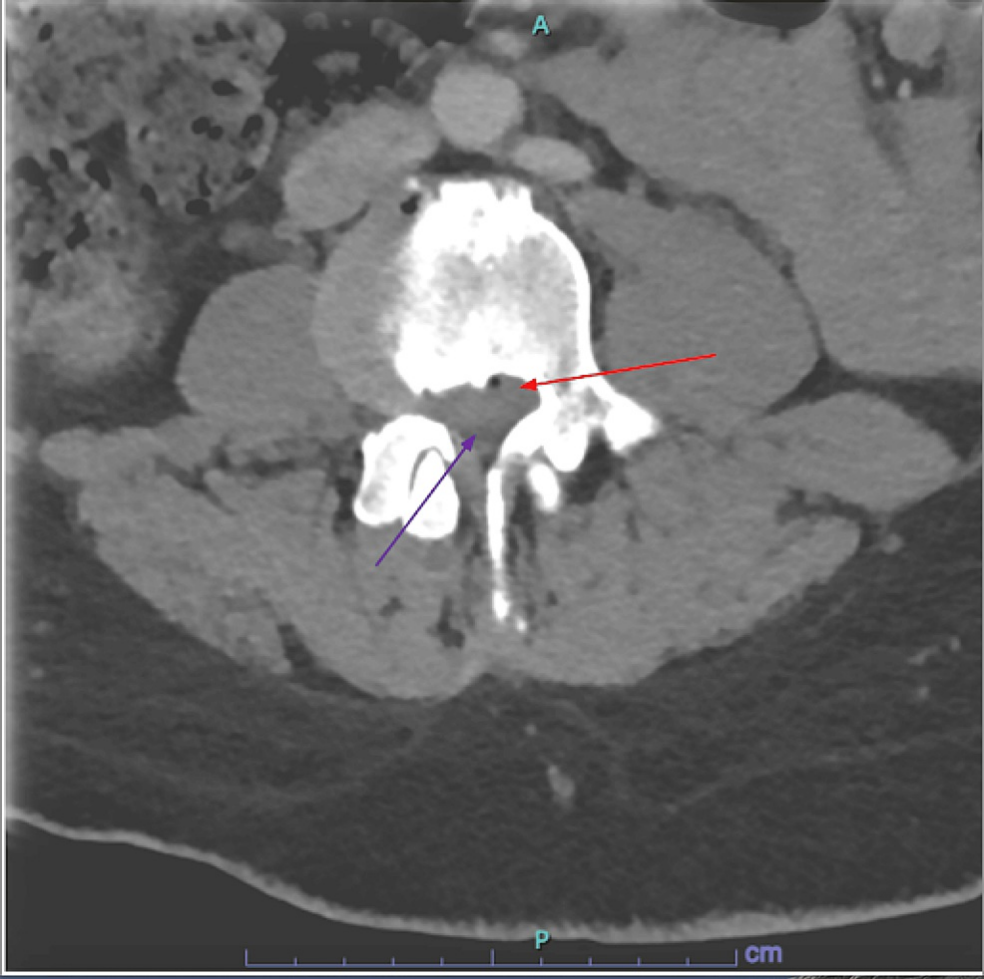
Emergency department computed tomography (CT) scan This image shows the patient's axial post-contrast CT scan in the ED, which showed a bulging disc noted by the light gray and denoted by the red arrow and a compressed spinal canal seen as dark gray and denoted by the purple arrow. The associated compressed nerves can be clinically correlated to the new-onset symptoms that are classic to cauda equina syndrome.
